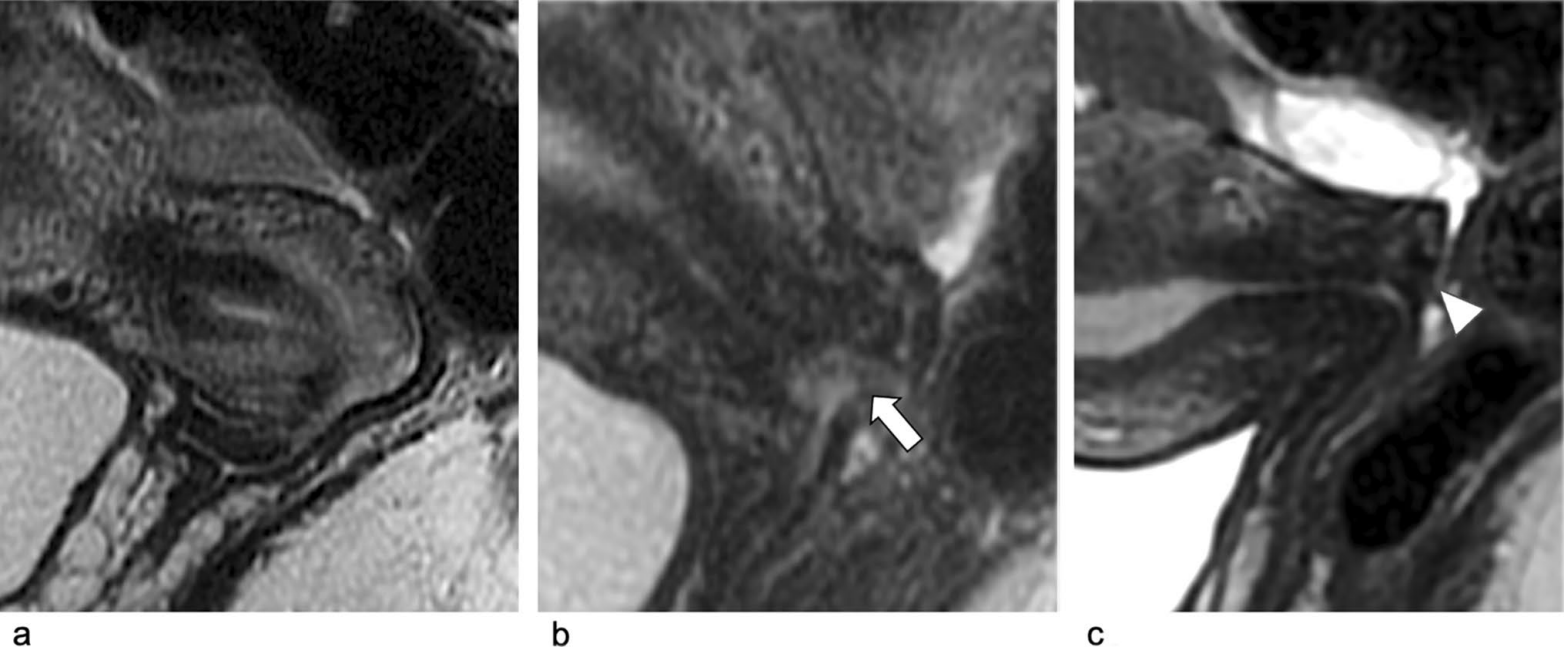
Post-conization MRI in a 36-year-old woman with cervical squamous cell carcinoma (FIGO IIA1), who underwent CKC after NACT. Sagittal T2-w images at baseline (a), after NACT (b) and after CKC (c). Baseline MRI shows the hyperintense cervical mass (a). Post-NACT shows reduction of the cervical lesion with persistence of a small hyperintense area within the posterior cervical lip (arrow in b), with diffusion restriction on DWI and ADC map (not shown). Post-conization sagittal T2-w images (c) show significant shortening of both anterior and posterior lips (arrowhead in c)
